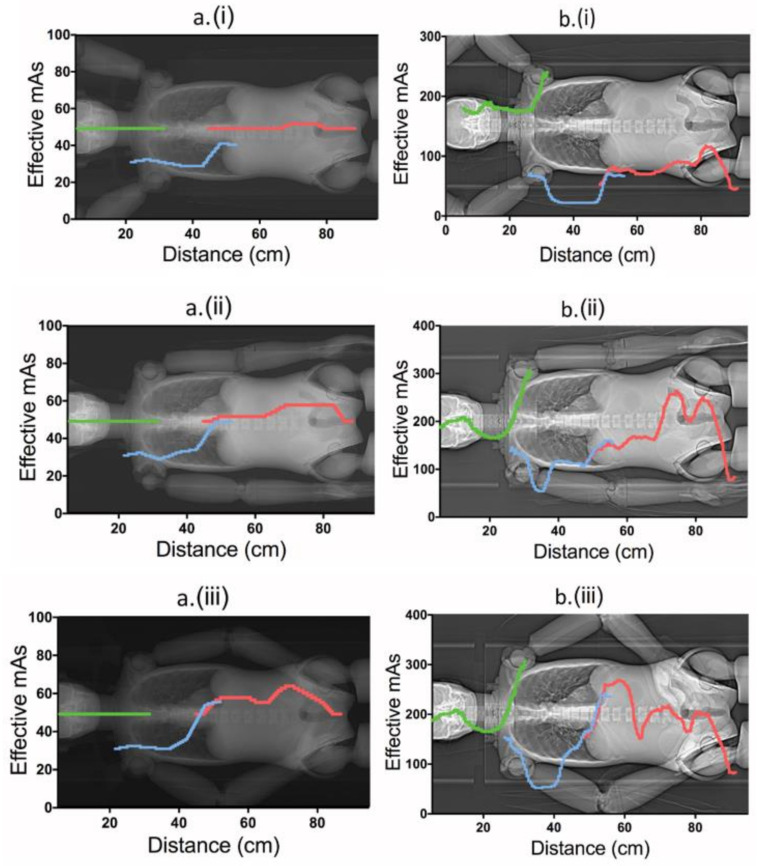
Effective mAs for the three different helical scans in different scenarios of arm positioning in the localizer radiographs (using corresponding protocol at both CT systems): (i) arms raised above the head, (ii) arms lowered along the trunk and (iii) arms along the trunk with hands placed on the abdomen, for Canon Aquilion One ((a); left column) and Siemens Definition AS ((b); right column).
